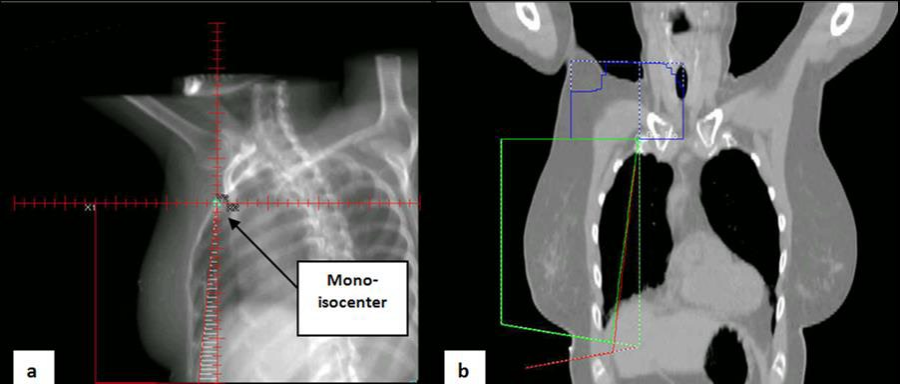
Three‐field setup using single‐isocenter technique. (a) DRR of the coronal BEV showing the position of the isocenter at a depth of 3 cm. Half‐beam blocked fields were used for the tangents and SCV. The MLC was used to mimic the two‐isocentric technique fields to reduce the volume of lung included in the treatment. (b) The coronal view of the planning CT showing the match between the SCV field (blue) and tangent field borders (red and green). For the supraclavicular field, the area outside the solid blue line represents areas shielded by the MLCs.
